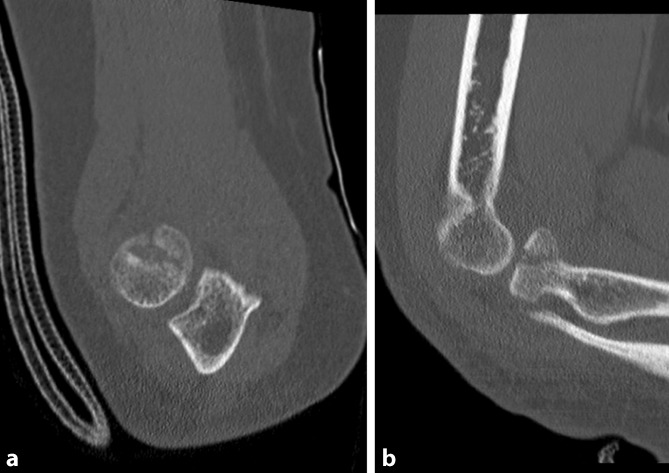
Computed tomography scans of a radial head fracture (Mason II)
